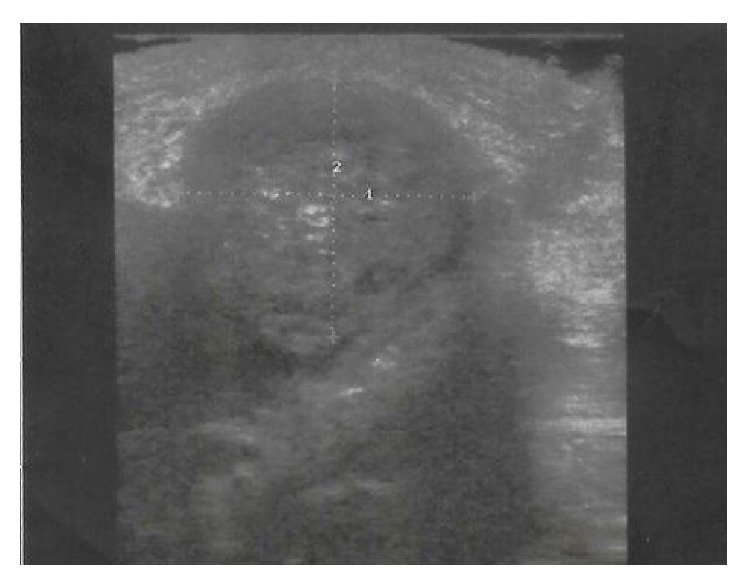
The ultrasound performed, gray scale mode B of the cervical mass. Solbiati index is L/T, respectively, *D*2/*D*1 = 23.6 mm/26.9 mm = 0.87.
